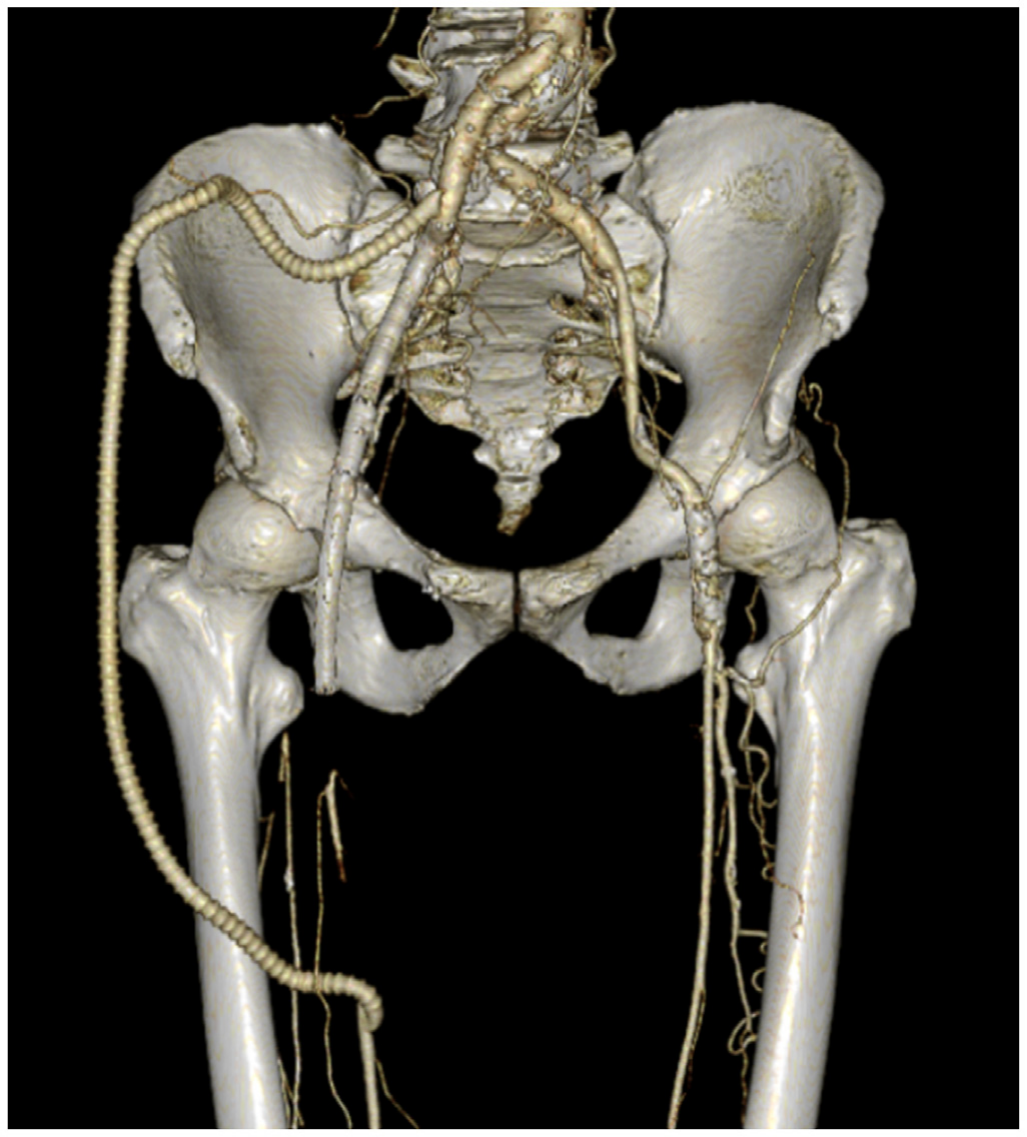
Postoperative reconstructed three-dimensional computed tomography angiogram showing successful revascularization achieved by lateral femoral bypass.
